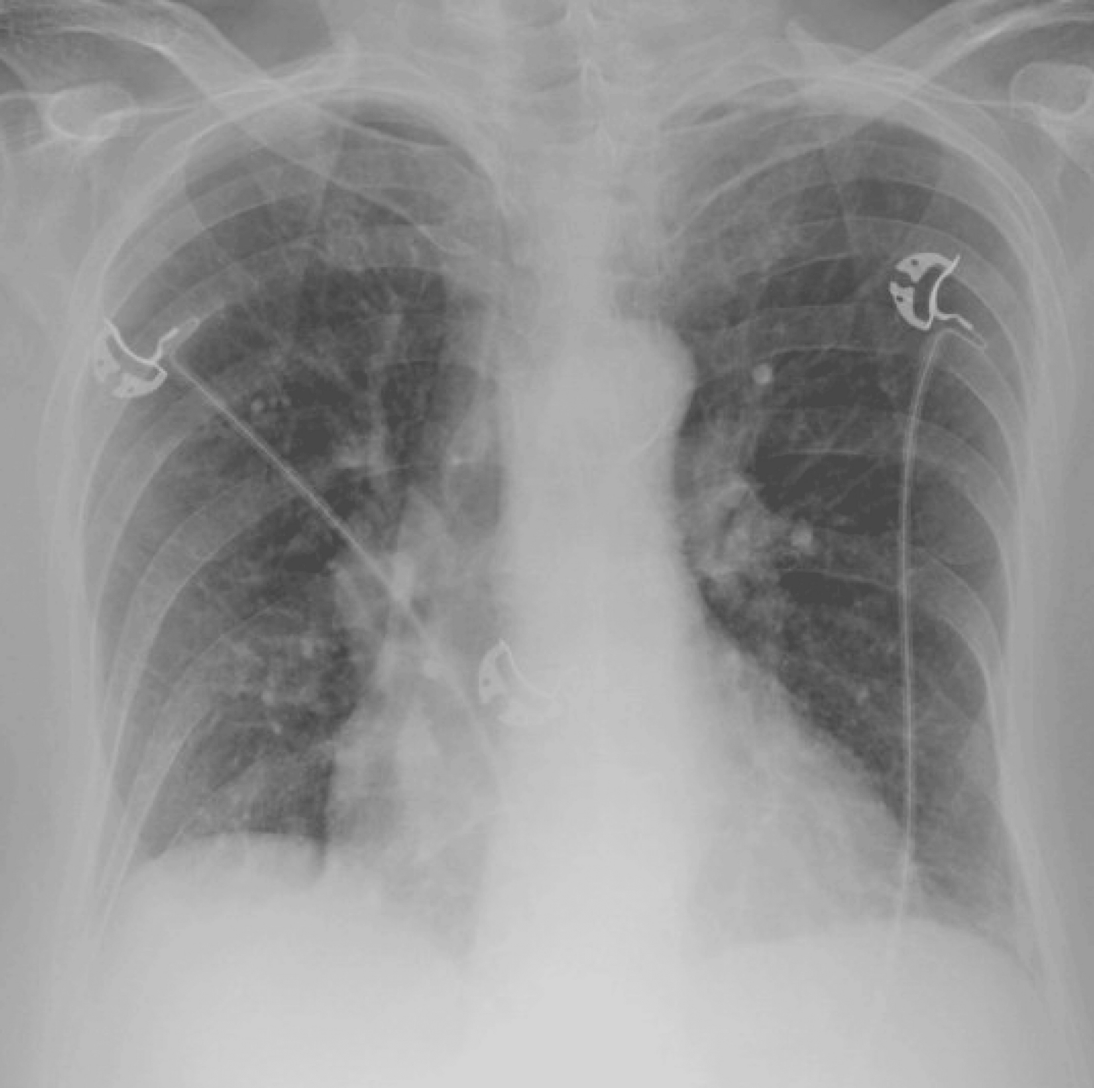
Chest radiograph on day 1 of hospitalization demonstrated mild bilateral infiltrates.
